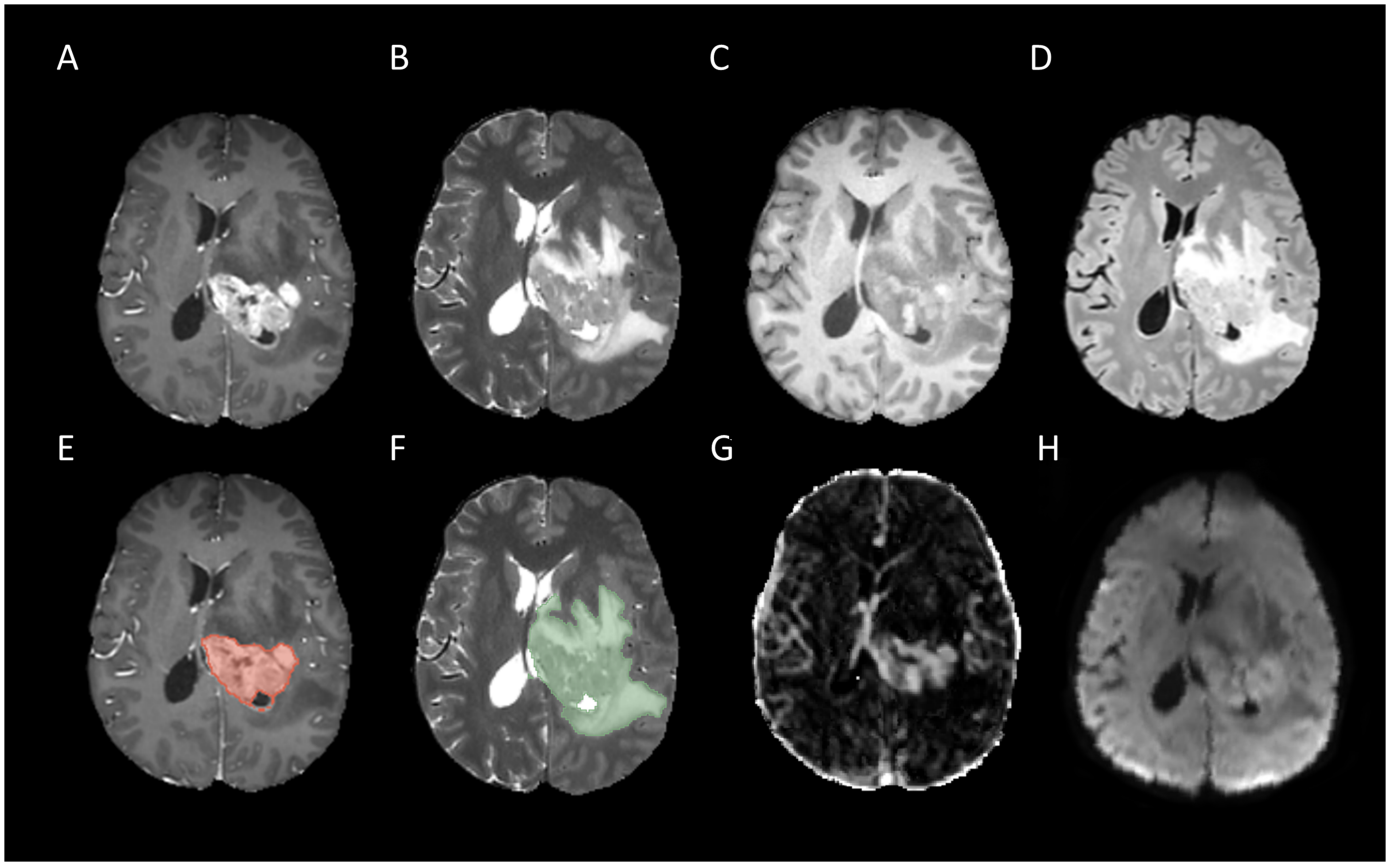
Example for MR images of a patient with MGMT methylated: T1CE (A), T2WI (B), T1WI (C), FLAIR (D), tumor core segmentation on T1CE (E), whole tumor segmentation on T2WI (F), Ktrans map of DCE (G), and MD map of DTI (H).
